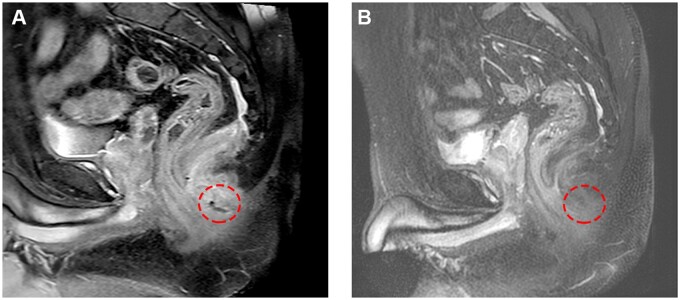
MRI images of a patient (Patient 4) with a fistula before (A) and after (B) 6 months of exosome injection reveal that the tract has been resolved
